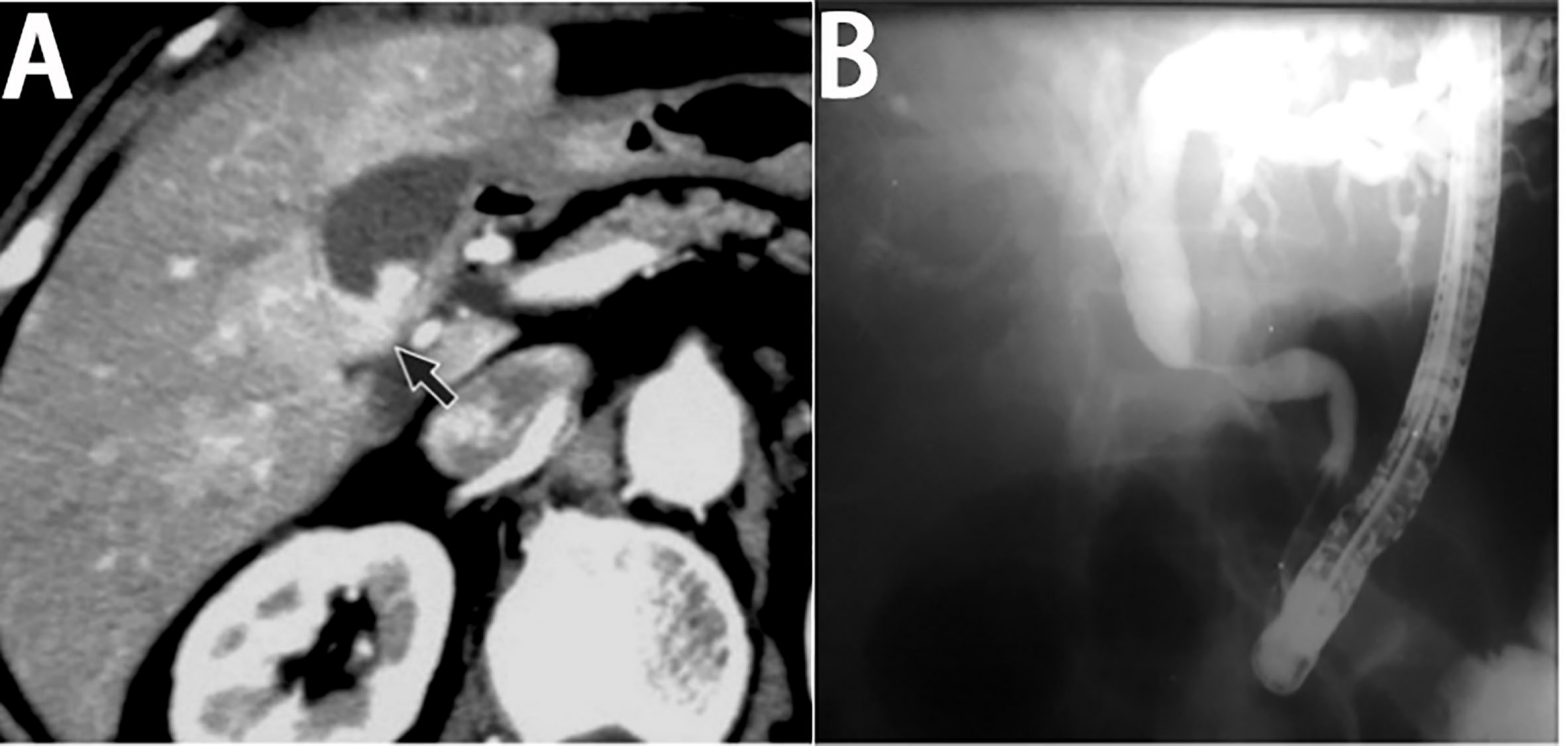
Imaging finding of primary biliary lymphoma. (A) On enhanced CT, the lesion of primary gallbladder lymphoma shows laminar enhancement on the mucosal surface of the gallbladder wall (black arrow). (with the permission of BIR publication). (B) ERCP of primary bile duct lymphoma shows a segmental luminal narrowing of mid common bile duct with marked dilation of proximal bile duct (with the permission of Elsevier).
